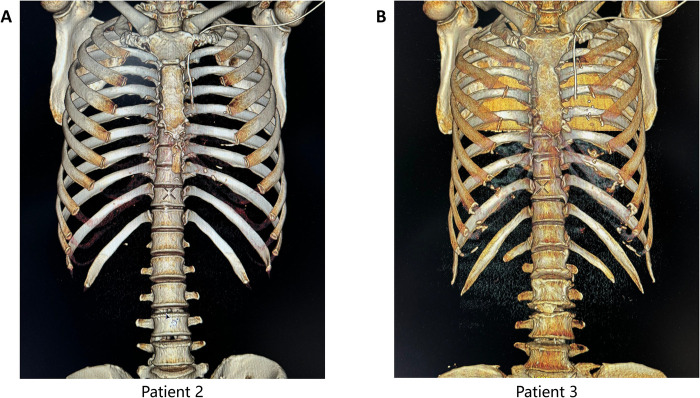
Postoperative 3D CT reconstruction of PLSVC patients.
